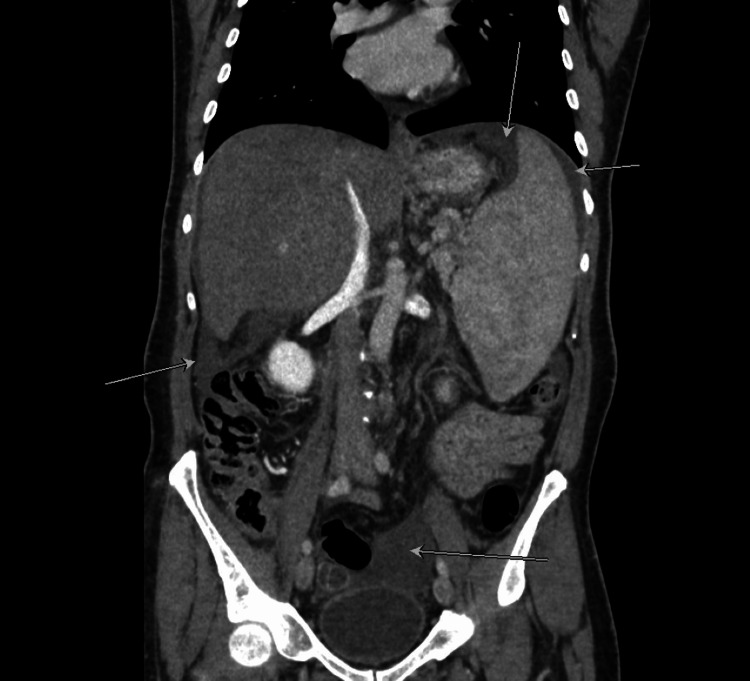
Contrast-enhanced abdominal CT revealed the accumulation of free fluid (ascites), highlighted by the white arrows
